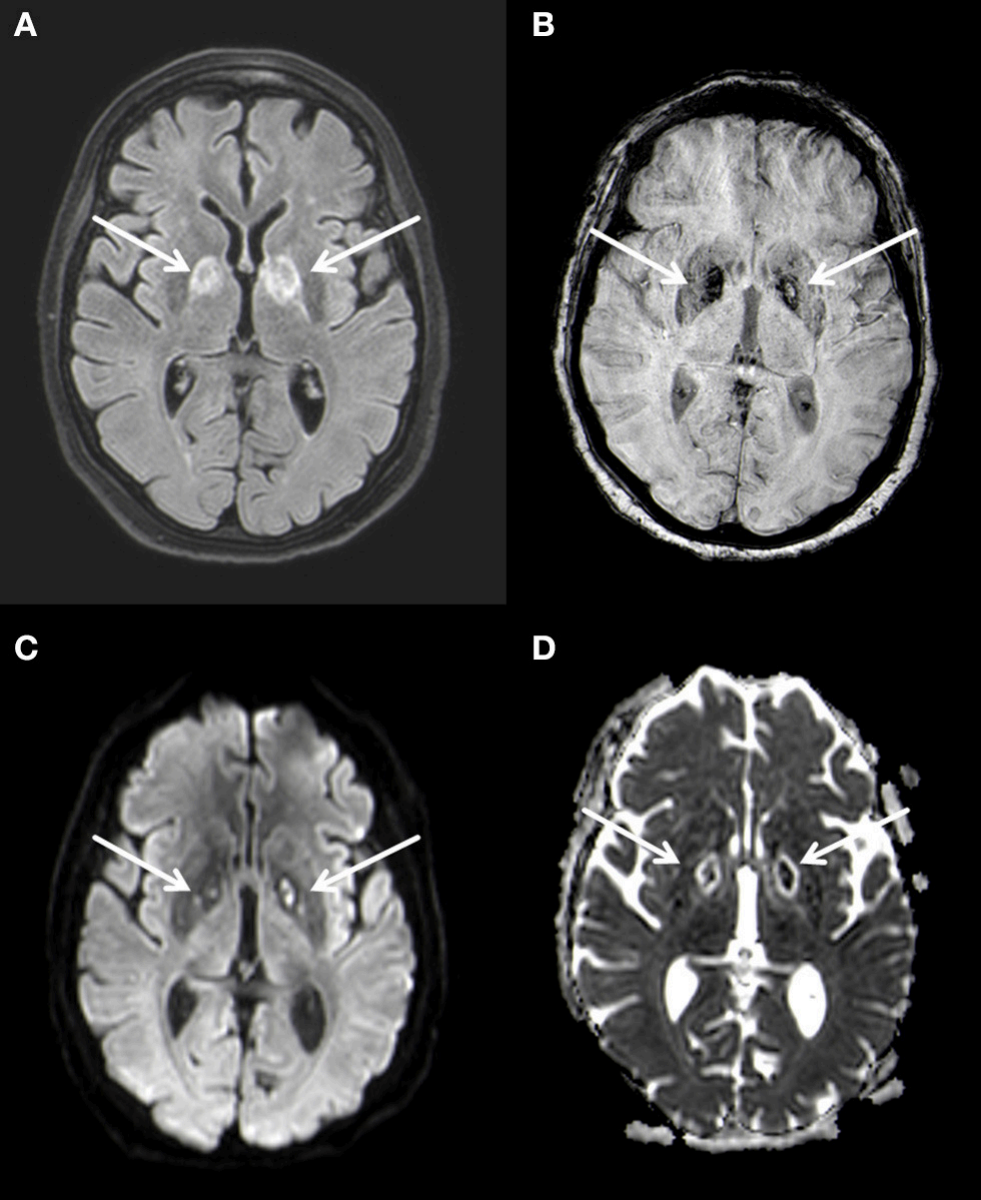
Bilateral basal ganglia necrosis with T2w hyperintense alterations (A) and hemoside deposits (B). These changes are diffusion-disturbed (C,D). 3 T Philips Ingenia, (A) FLAIR, (B) SWI, (C) b1000 image, (D) ADC map.
